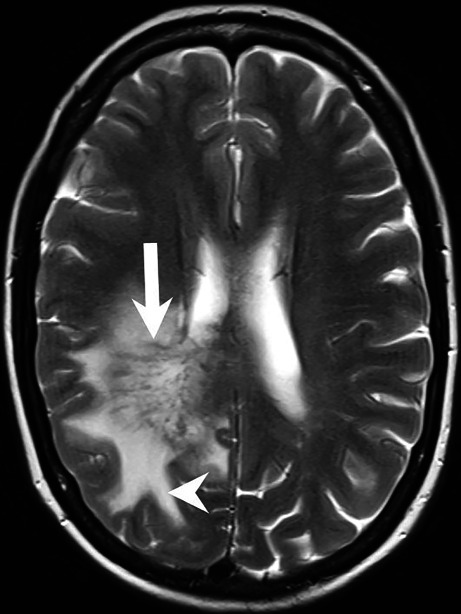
Axial T2-weighted images showed a lesion restricted to the white matter of the right frontoparietal lobe. The lesion consisted of hypointense linear structures radially connecting subependymal areas of the right lateral ventricle with the subcortical areas of the right frontal and parietal lobe (*arrow*). The lesion was surrounded by an extensive perifocal edema (*arrowhead*)
